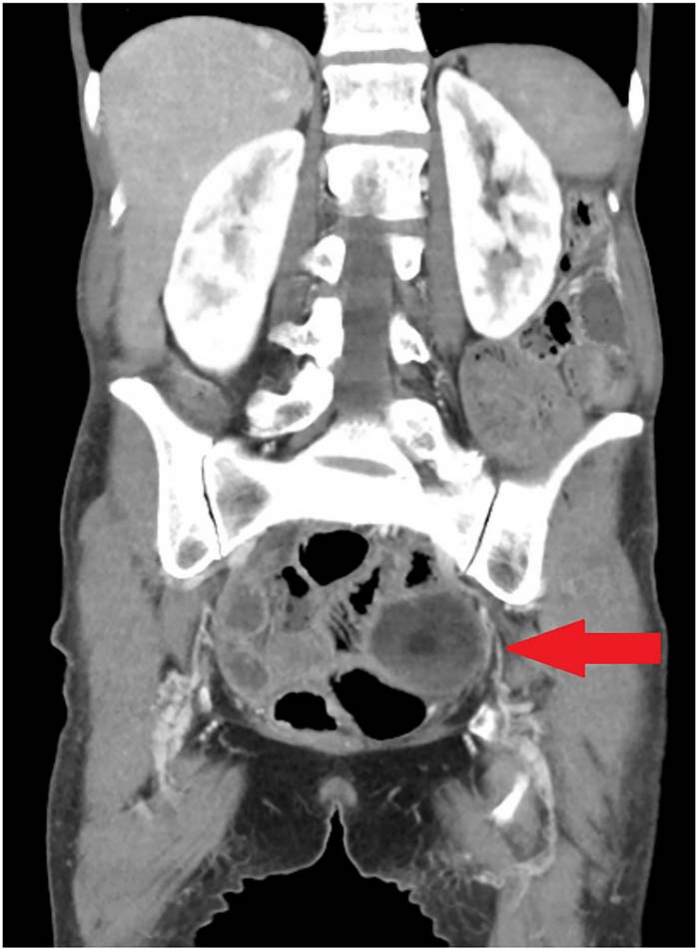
Coronal view of initial CT scan demonstrating left adnexal dermoid cyst (arrow).
